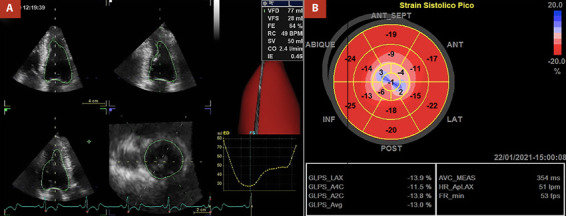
Determination of left ventricular (LV) systolic function parameters. Panel A shows a three-dimensional echocardiography image with normal LV volumes and ejection fraction. Panel B displays an LV global longitudinal strain map with altered myocardial deformation in the hypertrophic LV segments
